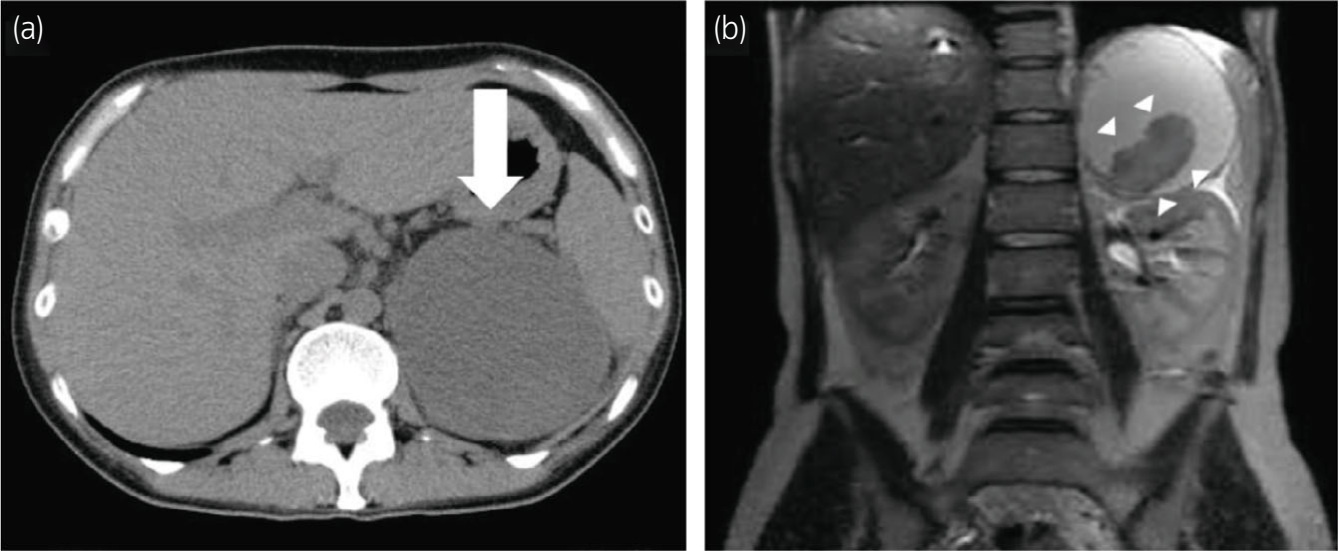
(a) CT image showing a 95 × 80 mm sized cyst on the rostral side of the kidney, and the inside of the cyst was almost homogeneous and water dense (➡). (b) T2‐weighted magnetic resonance image showing high signal with a 52‐mm internal tumor and septal wall (▵).
